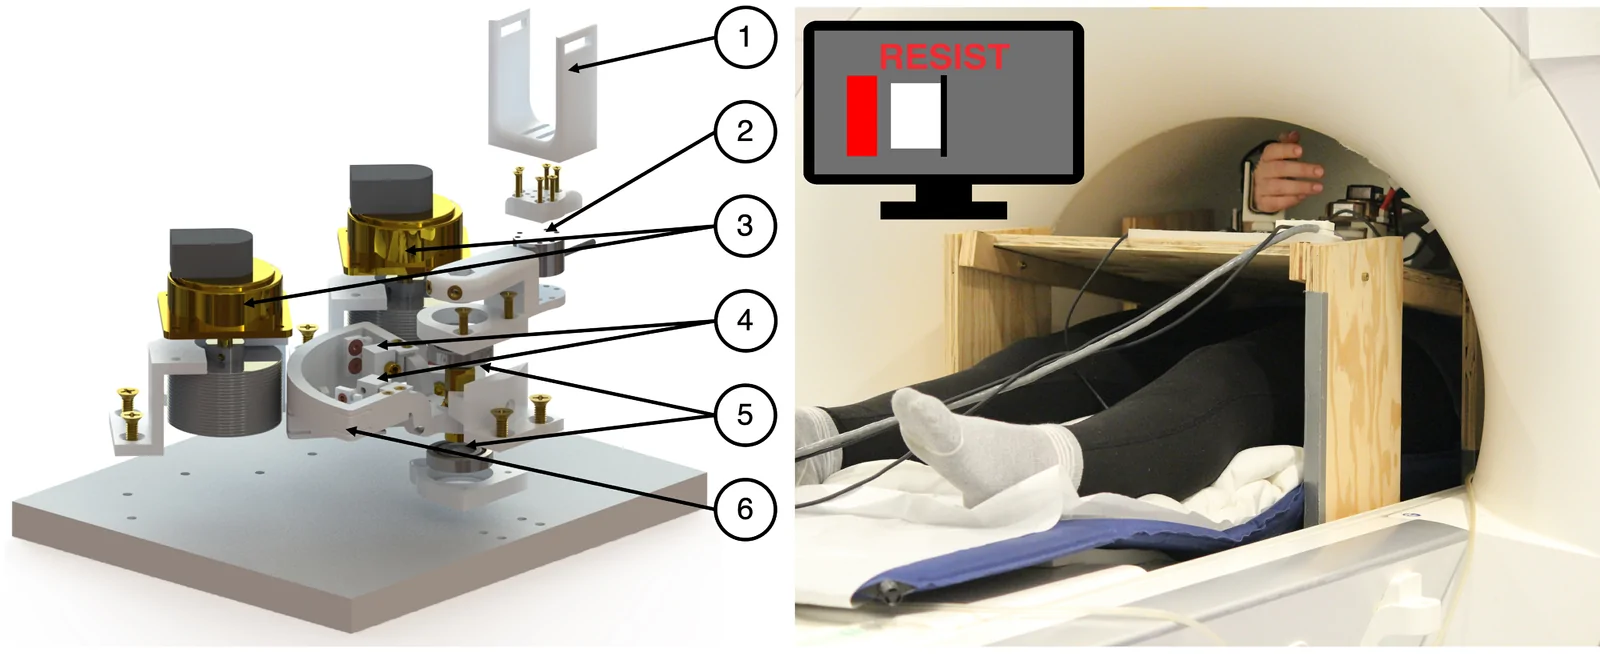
(Left) Exploded view of the modeled Dual Motor StretchWrist with labels for (1) hand support, (2) MR-compatible force–torque sensor, (3) MR-compatible piezoelectric ultrasonic motors, (4) tensioning mechanisms, (5) ceramic support bearings, and (6) capstan arc. (Right) A participant and the DMSW inside of the MRI scanner and a sample view of the Resist cued task.
